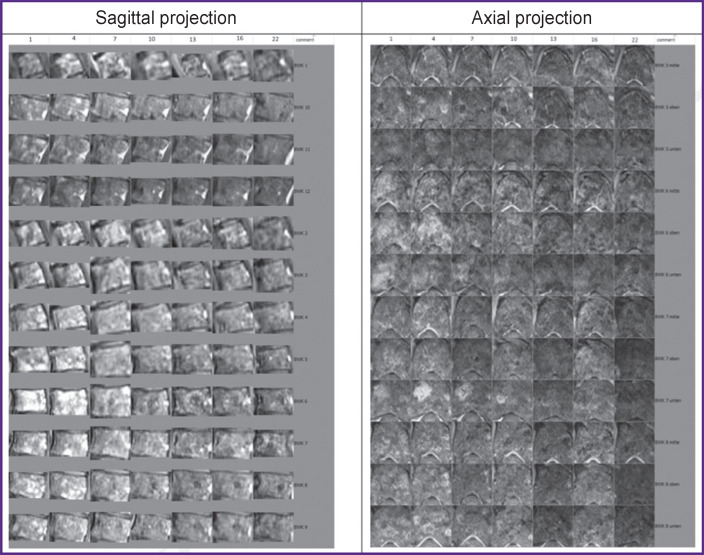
MRI in the T1-WI mode with contrast enhancement of the segmented thoracic vertebrae Th5–Th8 with a 3-month interval
